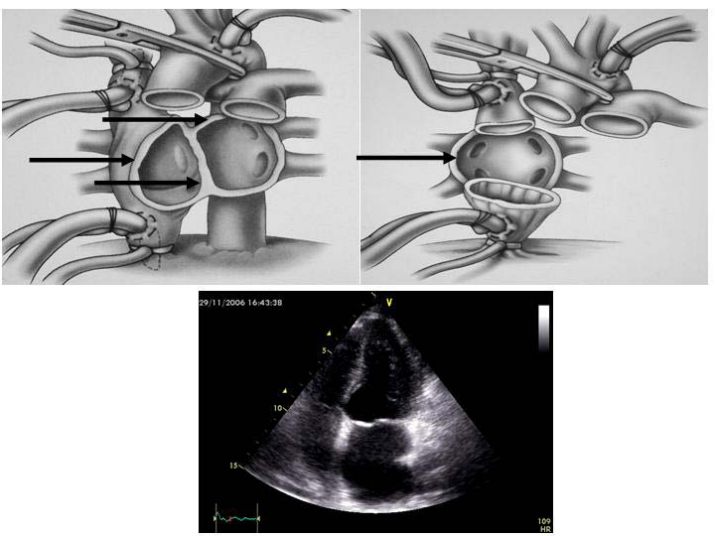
In the upper left panel a pericardial view (frontal) showing the edge of both atria which are preserved for conventional surgical technique (*Shumway technique*): possibility of interatrial ridge are dependent of the redundancy of donor as well as recipient residual tissue (arrows). In the upper right panel pericardial view (frontal) showing how the edge of both atria are removed for *bicaval surgical technique*: possibility of interatrial ridge are dependent of the redundancy of recipient septal residual tissue only (arrow). In the lower panel 2-D echocardiographic apical 4-chamber view showing left atrial enlargement and point of suture of a transplanted heart. Mod from Heart Transplantation – Churcill Livingstone – James K. Kirklin et al. 2002.
